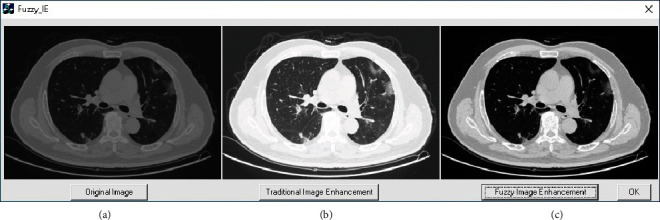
Contrast improvement of CT scan image (a) original image (b) enhanced image using fuzzy expected value.
